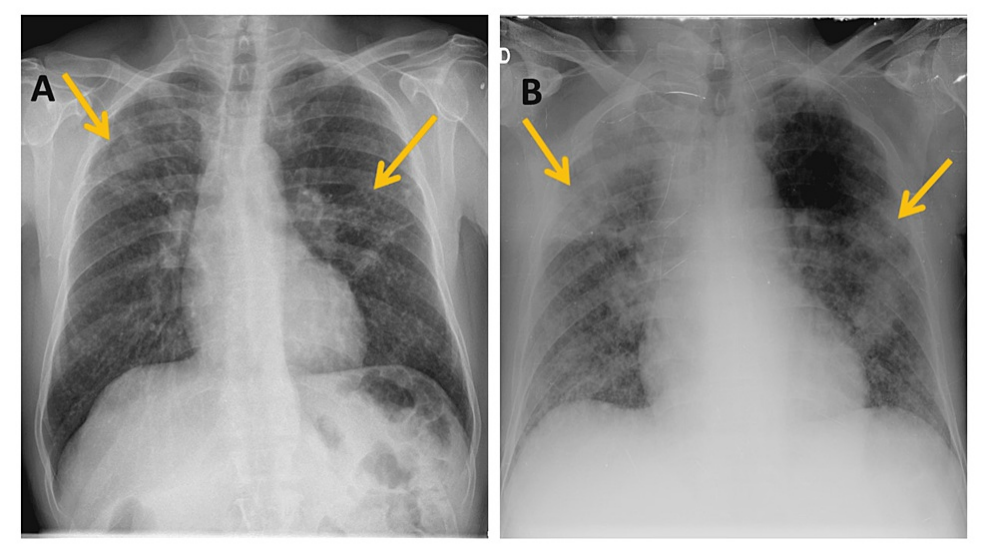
Chest X-rays A - Chest X-ray seven months before hospital admission; B - Chest X-ray in the emergency department, at admission, with worsening diffuse opacities compared to the previous ones (despite increased penetration) (arrows)
